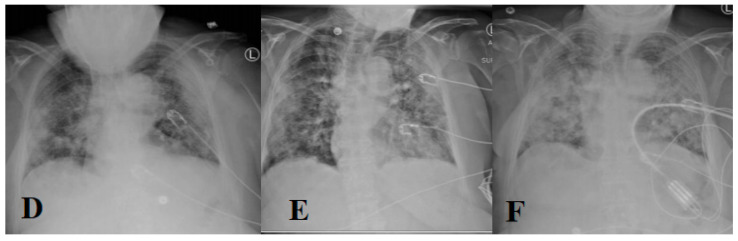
(A–F) subfigures show progression of an X-ray image for a COVID-19 patient across days (1, 3, 6, 7, 8, and 10, respectively).
